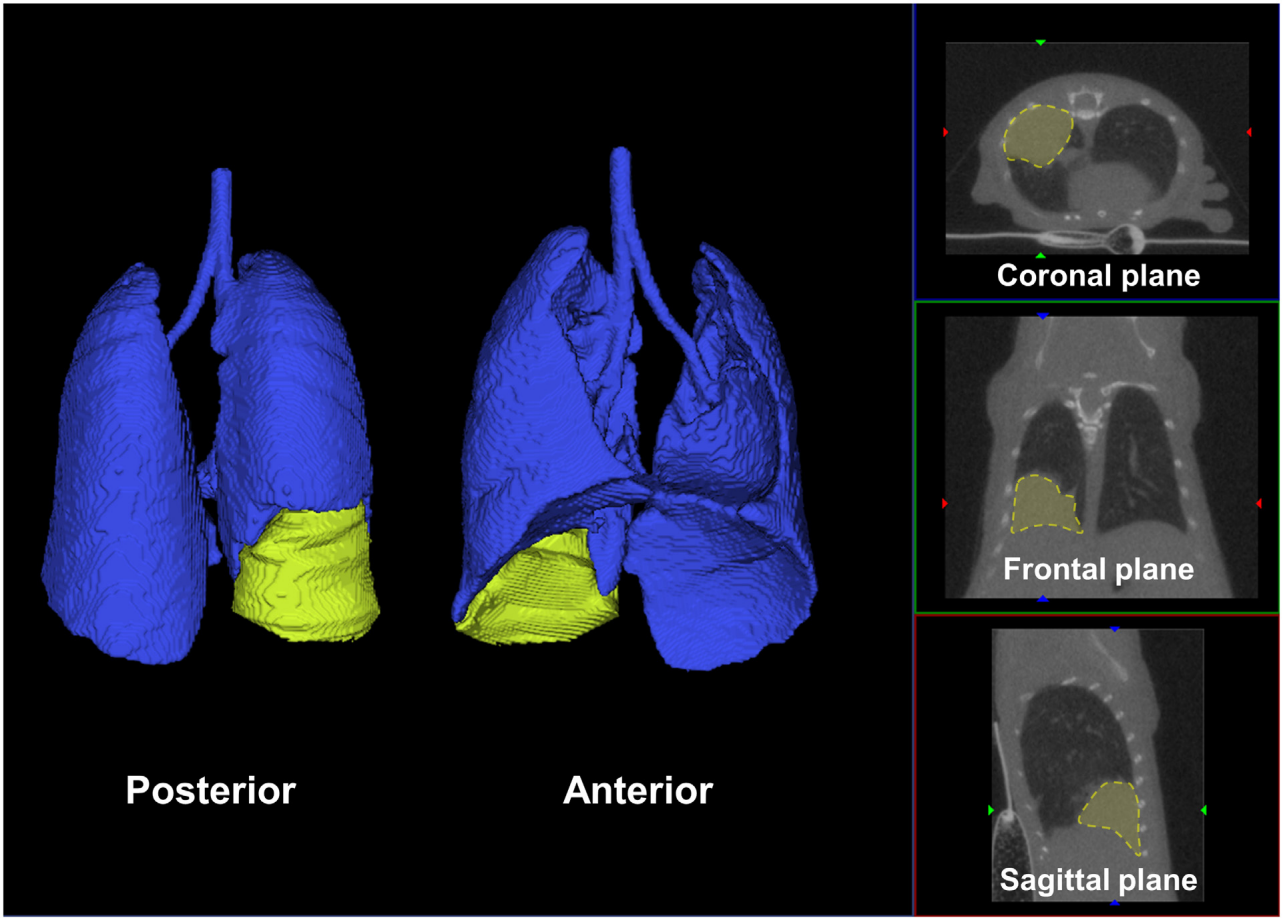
Assessment of tumor volumes with micro Computed Tomography. 3D rendering of CT scan anterior and posterior reconstructions, after processing the tumor delineation on 2D slices from coronal, frontal or sagittal plane. Healthy lung parenchyma is blue whereas tumor is highlighted in yellow.
